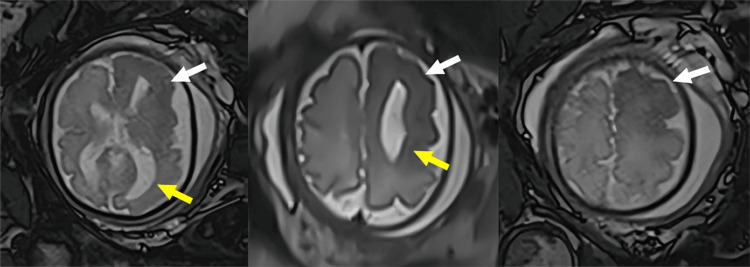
Fetal magnetic resonance imaging, T2 sequence, axial sections. Increased size of the left cerebral hemisphere, with dilatation of the left lateral ventricle (yellow arrow), alteration in corticosubcortical differentiation and hypointensity of the white matter (white arrow).
